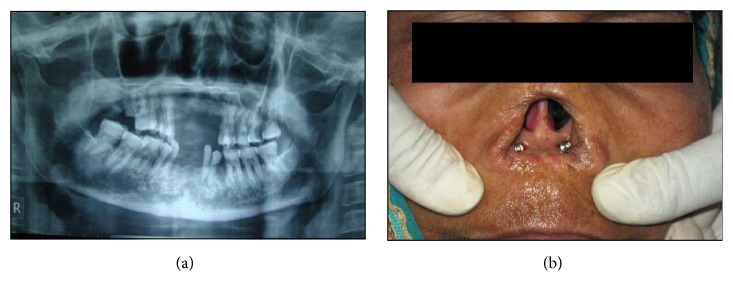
(a) Preoperative panoramic radiograph showing partially edentulous maxillary and mandibular arches. (b) Patient's face with O-ring post over implants in anterior nasal floor.
